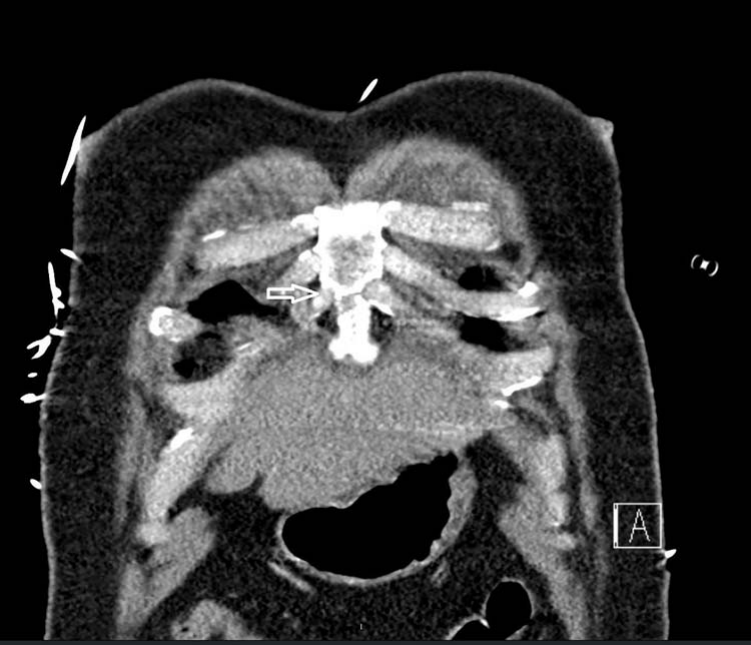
Computed tomography scan showing nondisplaced sternal body fracture (arrow).
